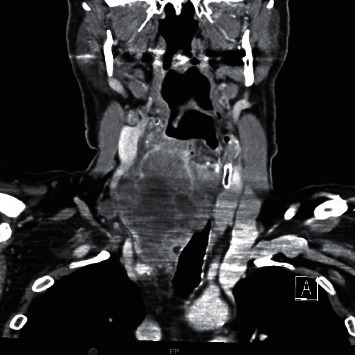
CT scan of the neck at presentation (coronal image).
